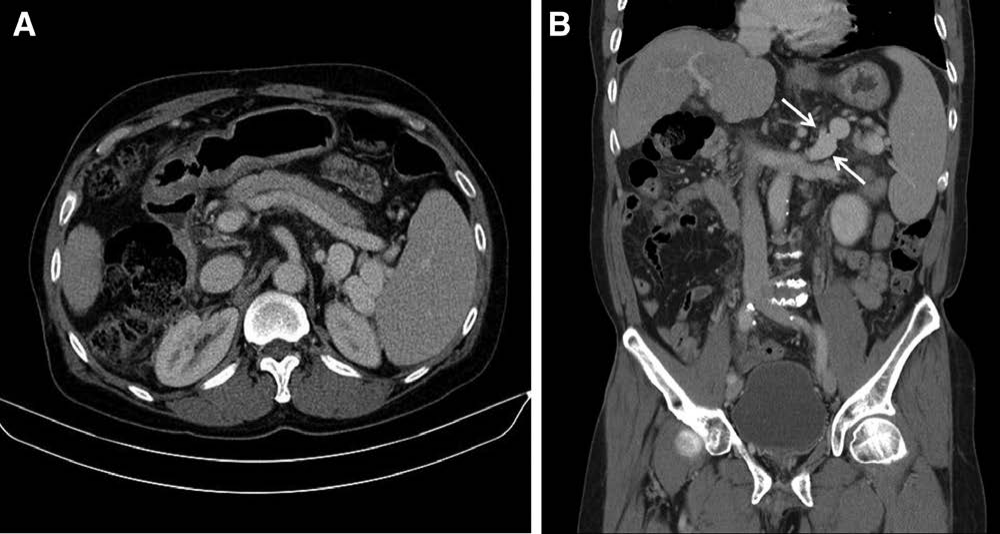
A: CT axial images showing collateral vessels around spleen. B: Two splenorenal shunts (arrows) are identified on CT coronal reconstruction images. CT = computed tomography.
